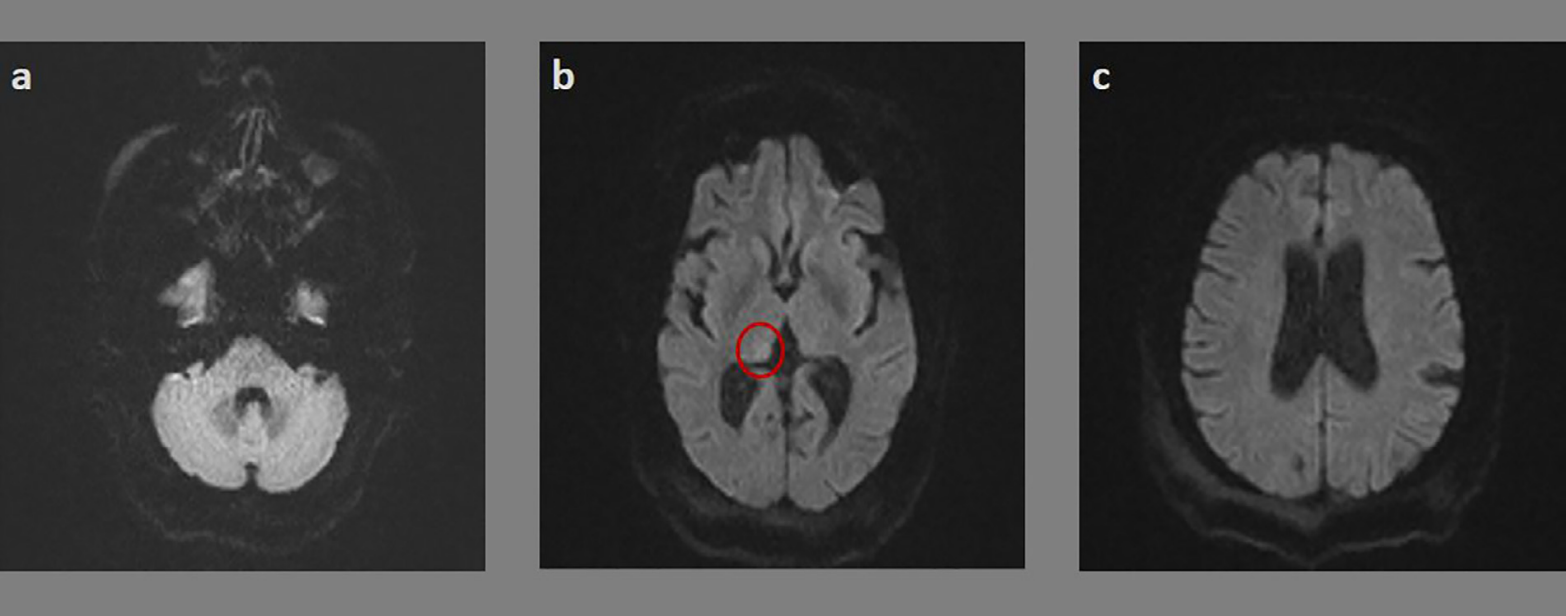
Follow-up brain DWI MRI revealing complete resolution of cerebral cortical and cerebellar signal changes (A, B, C), and reduced hyper-signal in the right pulvinar thalamic nuclei (red circle) (C) compared to the prior images. (Color version of figure is available online.)
